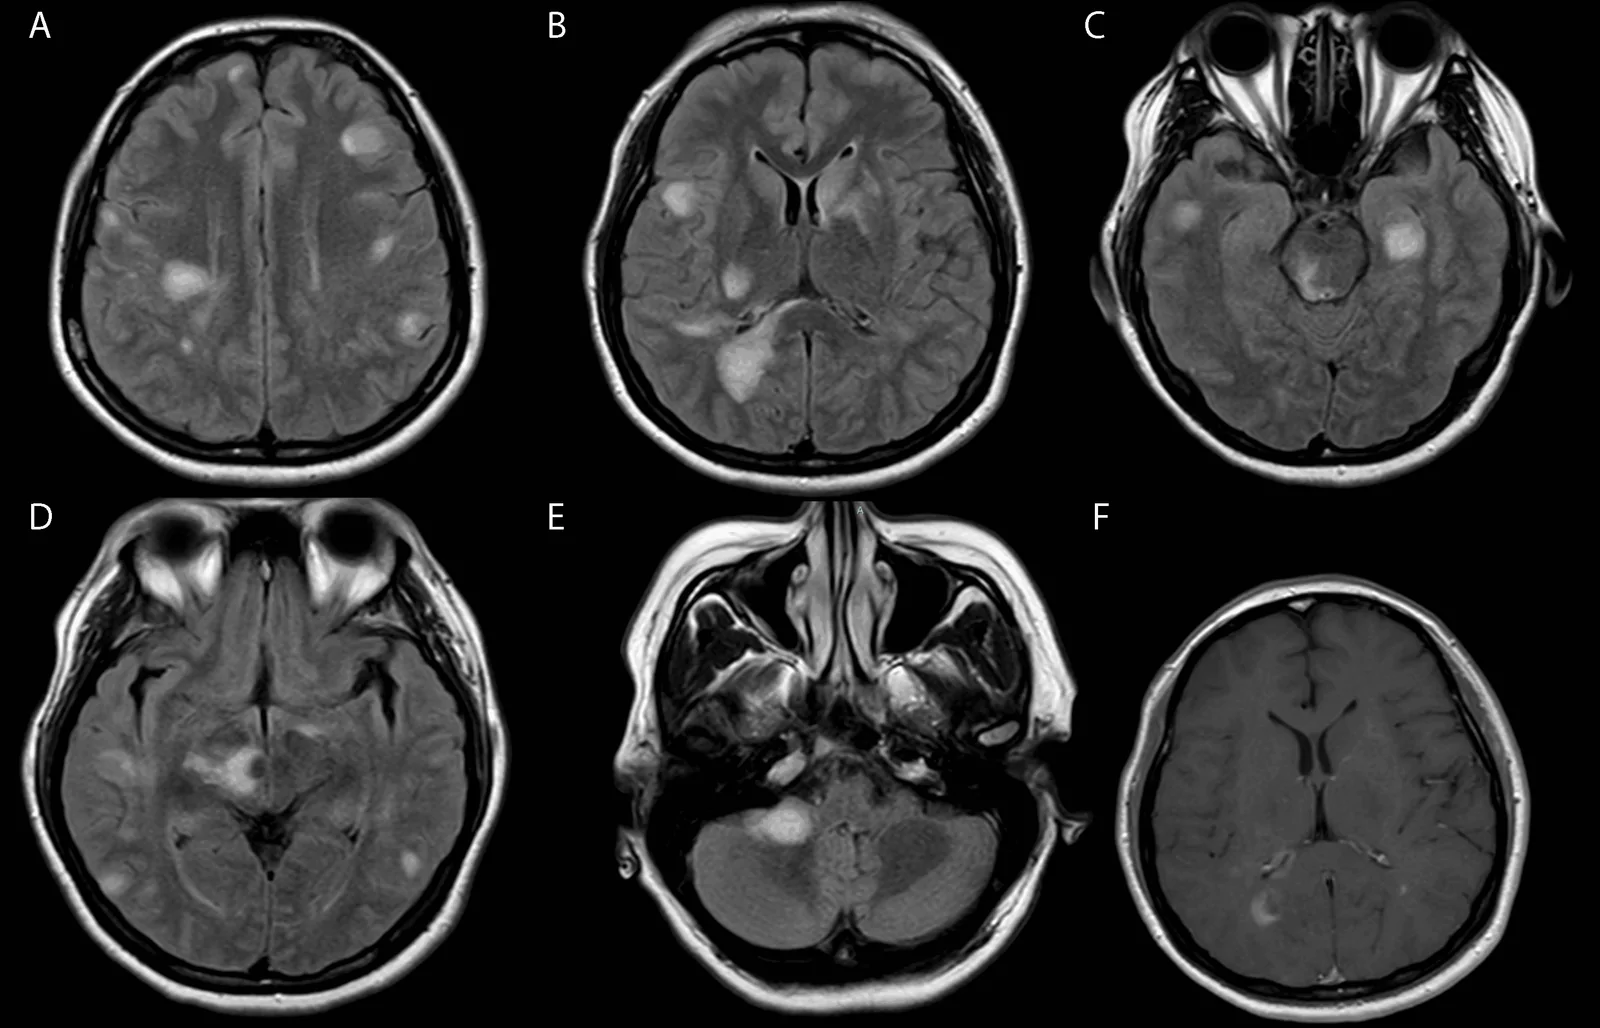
Panel showing white matter lesions on MRI brain T2 FLAIR MRI brain images showing white matter lesions consistent with demyelination, which are subcortical (A and B), subcortical and pontine (C), midbrain and subcortical (D), and cerebellar (E). Enhancement of the parietal lobe lesion is seen in the T1 postcontrast image (F). These large and poorly marginated lesions involving subcortical white matter, brainstem, and cerebellum are characteristic of an ADEM-like/MOGAD phenotype and distinct from the ovoid periventricular lesions typical of MS. Images were obtained on day 96 after initial COVID-19 vaccination. ADEM: Acute disseminated encephalomyelitis; MOGAD: myelin oligodendrocyte glycoprotein antibody-associated disease
